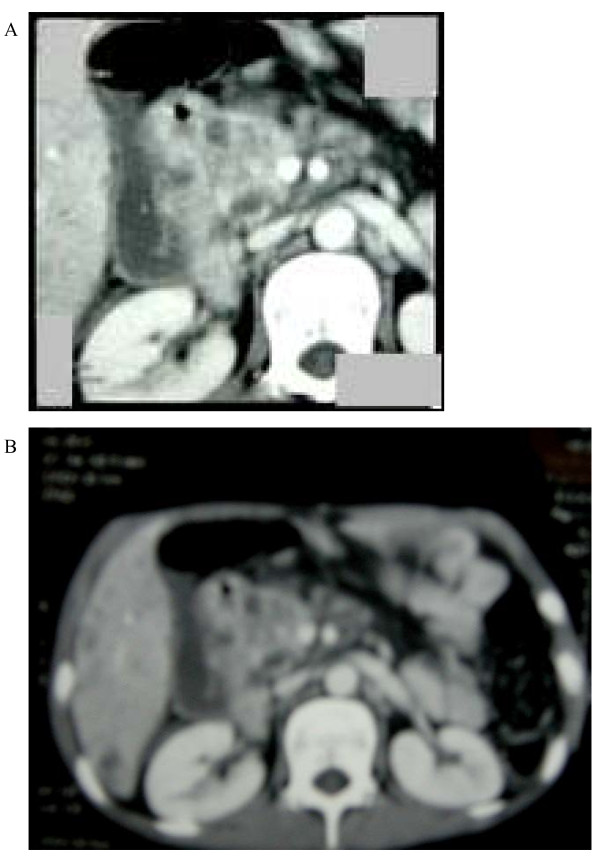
Metastatic pancreatic adenocarcinoma in our 13-year-old patient. A computerized tomography (CT) scan of the abdomen showed a tissular heterogeneous tumor of the head of the pancreas measuring 49 mm × 37 mm.
